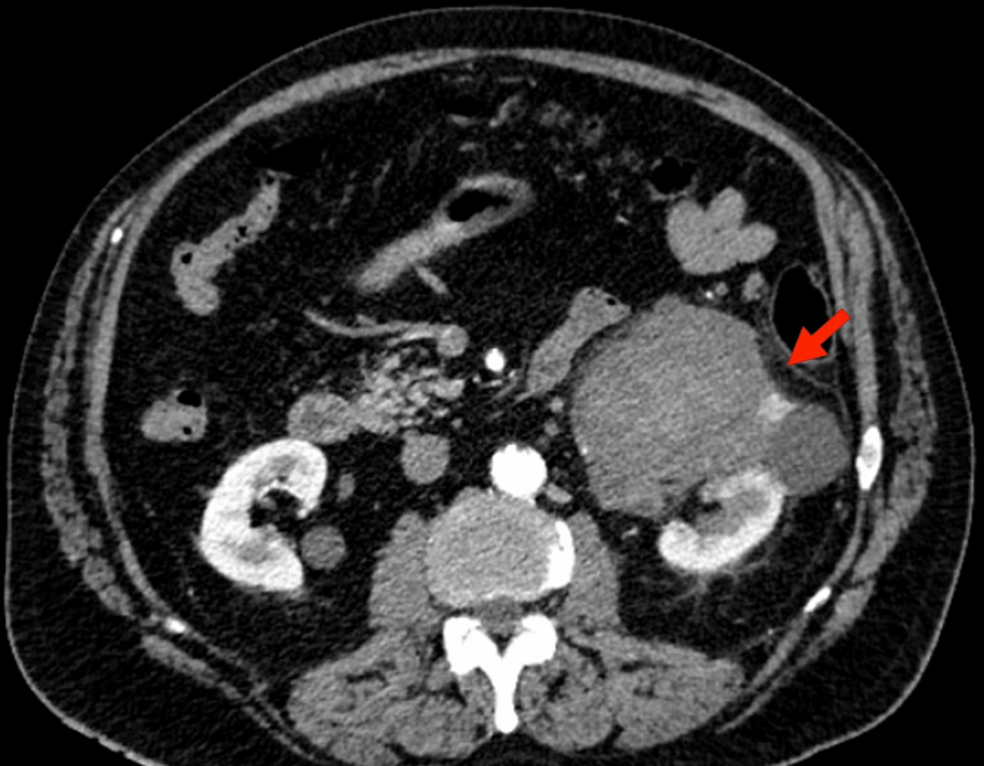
CT scan axial view at presentation CT scan axial view showing a large hypodense mass in the left kidney (red arrow). CT: Computed tomography
